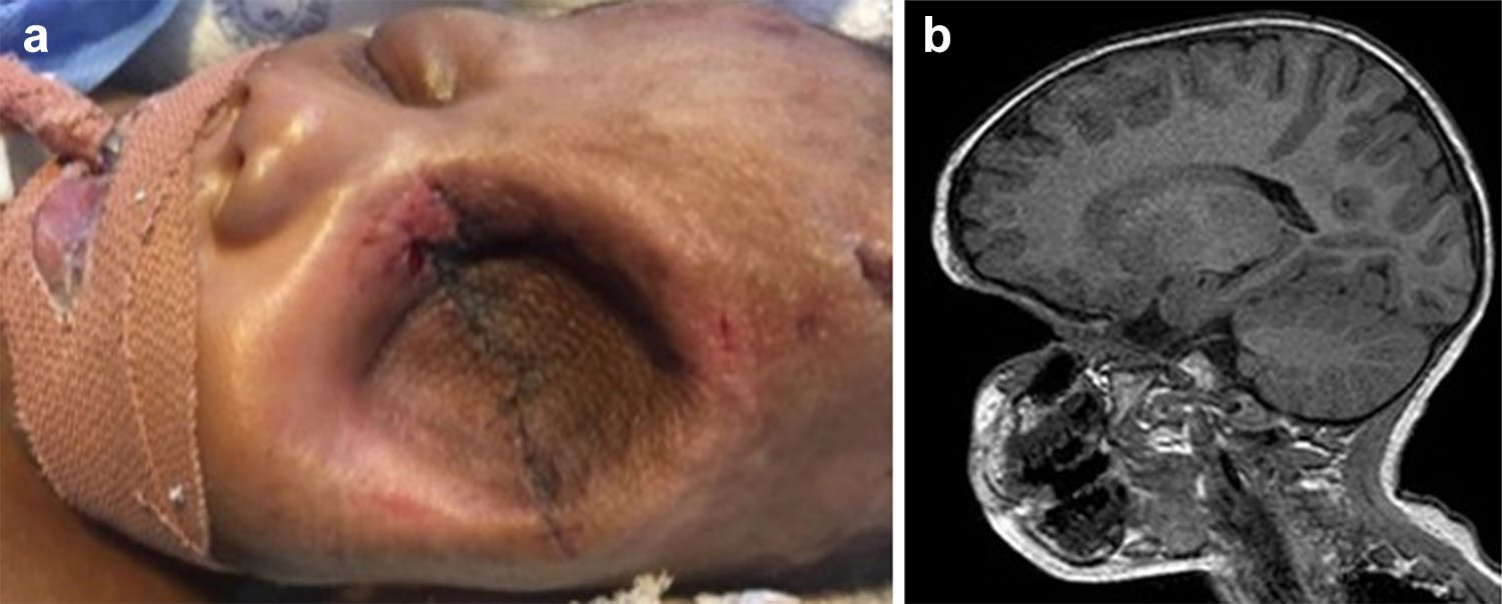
a The eyelids were then sutured closed by the ophthalmologist and a small dressing placed over the incision site. b The residual mass was demonstrated, comprising of a small intra-canalicular (8 × 5 mm) and intracranial (13 × 8 mm) component, markedly decreased compared to the initial MRI
